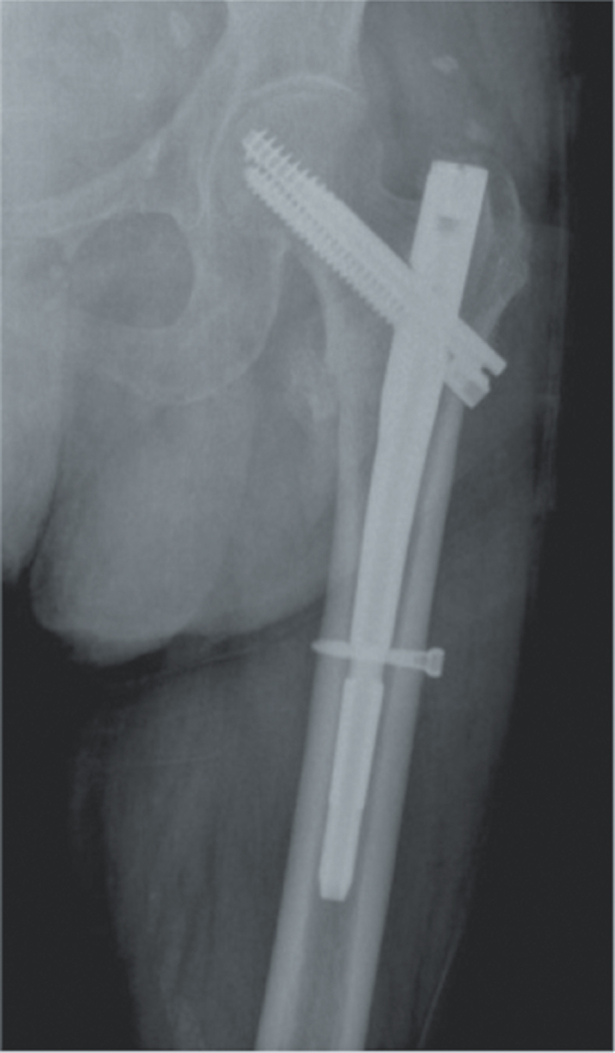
X-Ray view of the antegrade intertrochanteric nail (AIN).
